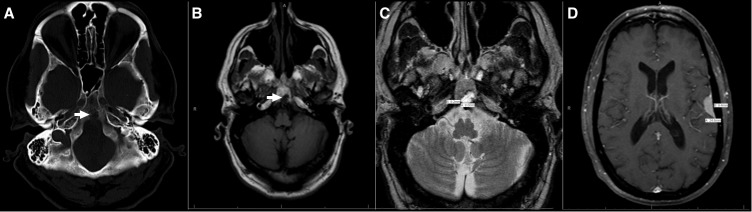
Brain Radiography for Patient 1 (A) Axial CT (bone window);* *(B) T1 Axial MRI; (C) T2 Axial MRI; (D) Axial MRI
